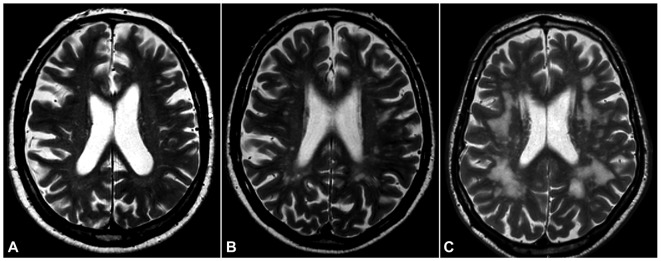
White matter hyperintensities (WMHs). T2-weighted magnetic resonance imaging (MRI) of the brain in three of our subjects, showing three grades of severity according to the Leukoaraiosis And DISability (LADIS) visual scoring scale (modified Fazekas scale): (A), mild; (B), moderate; (C), severe.
